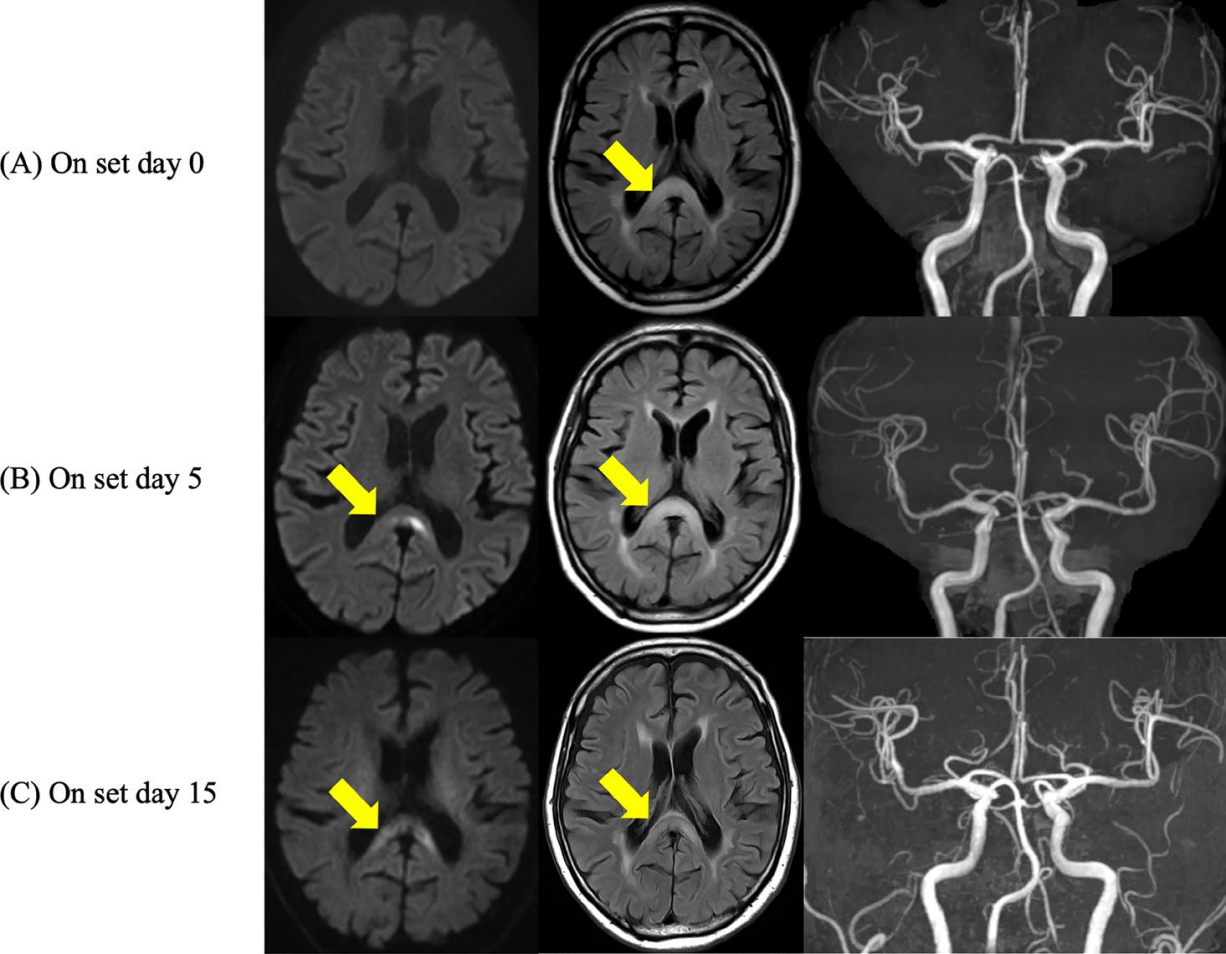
Brain MRI in this case. (A) Day 0: faint splenial hyperintensity. (B) Day 5: marked hyperintensity on FLAIR/DWI (arrows). (C) Day 15: decreased signal after thiamine (arrows).
